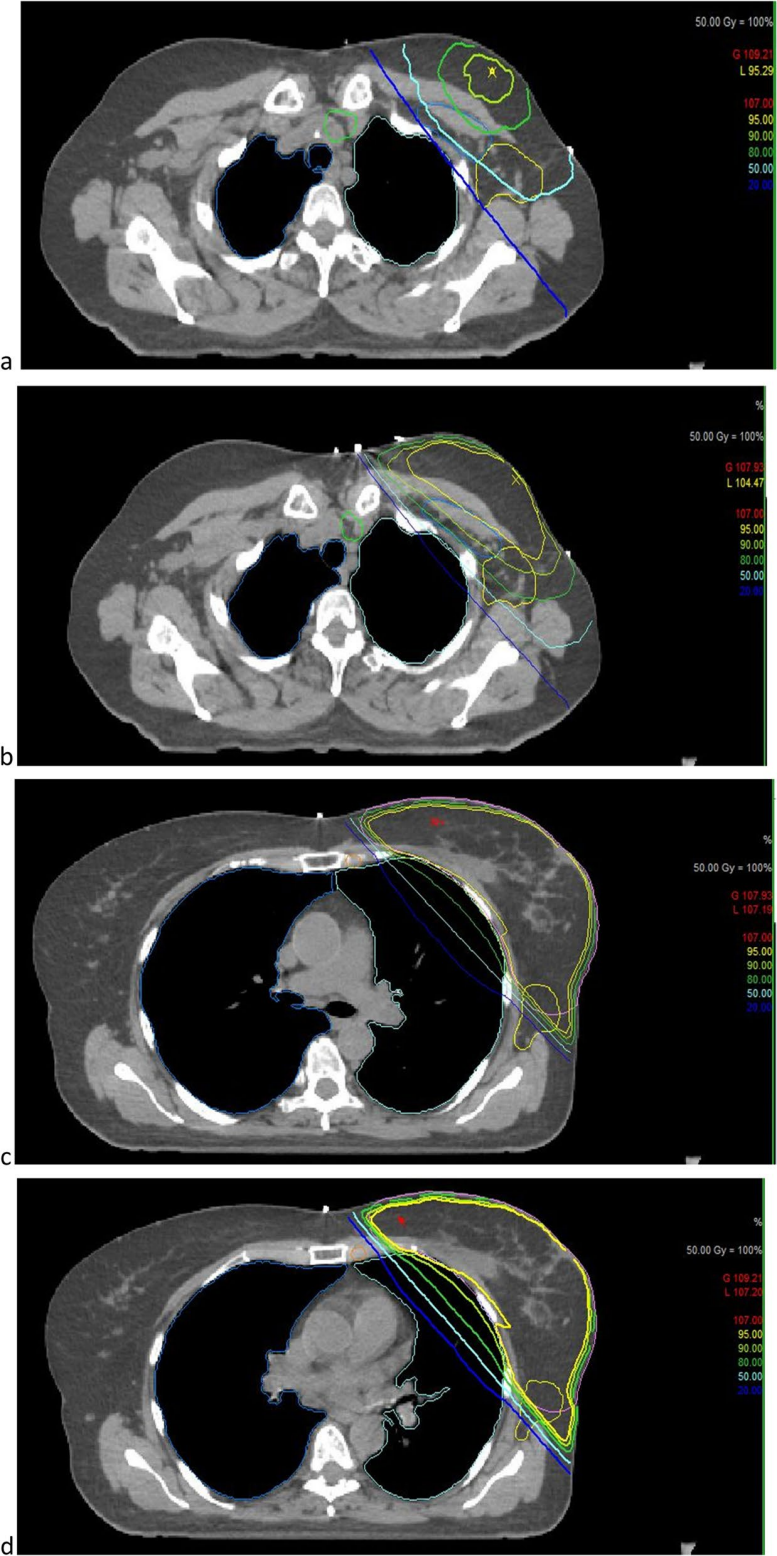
Delineation of locoregional lymph nodes and isodose distribution. a-c: Delineation of the supraclavicular (green), axillary lymph levels I (yellow) and II (light blue axillary lymph nodes in level III (blue), internal mammary (orange). a + c: CT scan in DIBH; b + d: CT scan in FB; a-d: Visualization of isodose distribution in DIBH and FB
